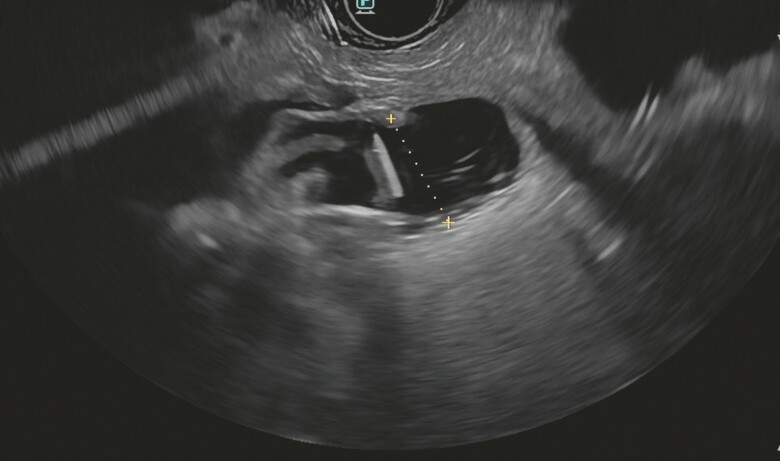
Endoscopic ultrasound image showing the instillation of contrast and saline with methylene blue via a nasojejunostomy tube at the level of the efferent loop of the anastomosis, with correct positioning being confirmed by the aspiration of methylene blue with a 19G needle.
